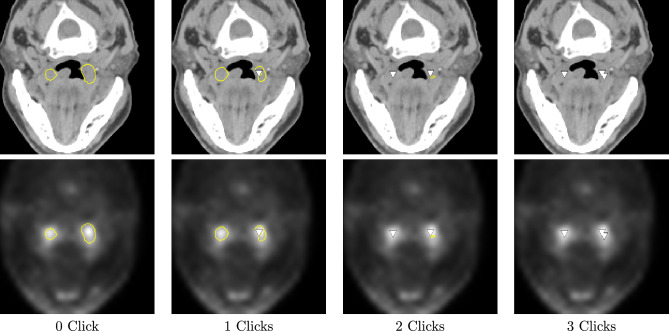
The progressive refinement of segmentation of 2S-ICR shown for the first three interactions overlaid on CT (top row) and PET (bottom row) slices. In addition, false positives are marked in yellow and clicks with white arrow.
